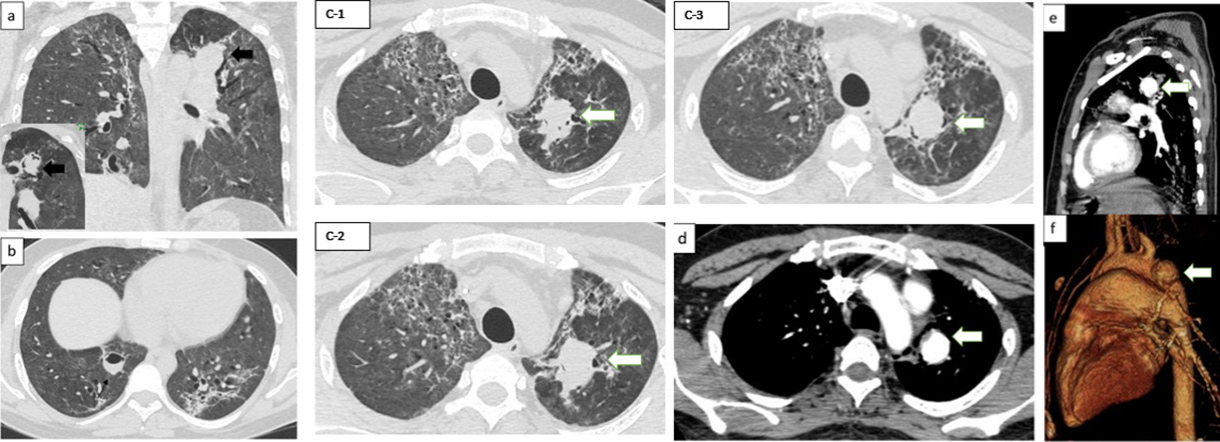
Pulmonary artery pseudoaneurysm. coronal (a) and serial axial (c-1,c-2,c-3) HRCT images show a focal zone of consolidation (white block arrows) in the left upper lobe. It is also seen abutting the left upper lobe bronchus on MiniP coronal image (inlet image in Figure 3a). Axial image of a lower section (b) shows partial resolution in the size of the fungal cavity. Axial sections of the pulmonary angiogram (d) Sagittal reformatted MIP image (e) and Sagittal 3D VRT image (f) shows the pseudoaneurysm communicating with the segmental branch of the left upper lobar artery.
